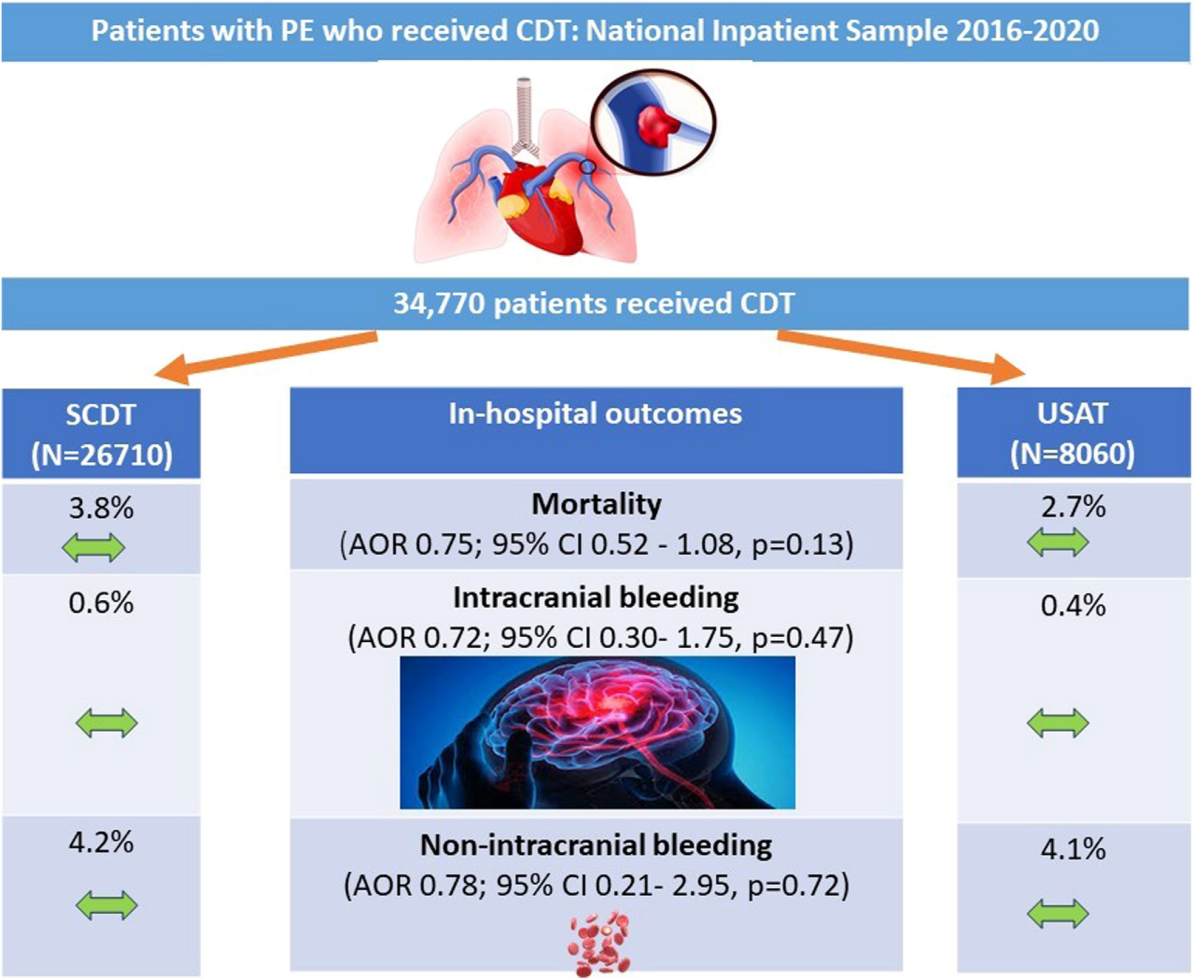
In-hospital outcomes with standard catheter-directed thrombolysis (SCDT) vs ultrasound-assisted thrombolysis (USAT) for acute PE. AOR, adjusted odds ratio; CDT, catheter-directed thrombolysis; PE, pulmonary embolism.
